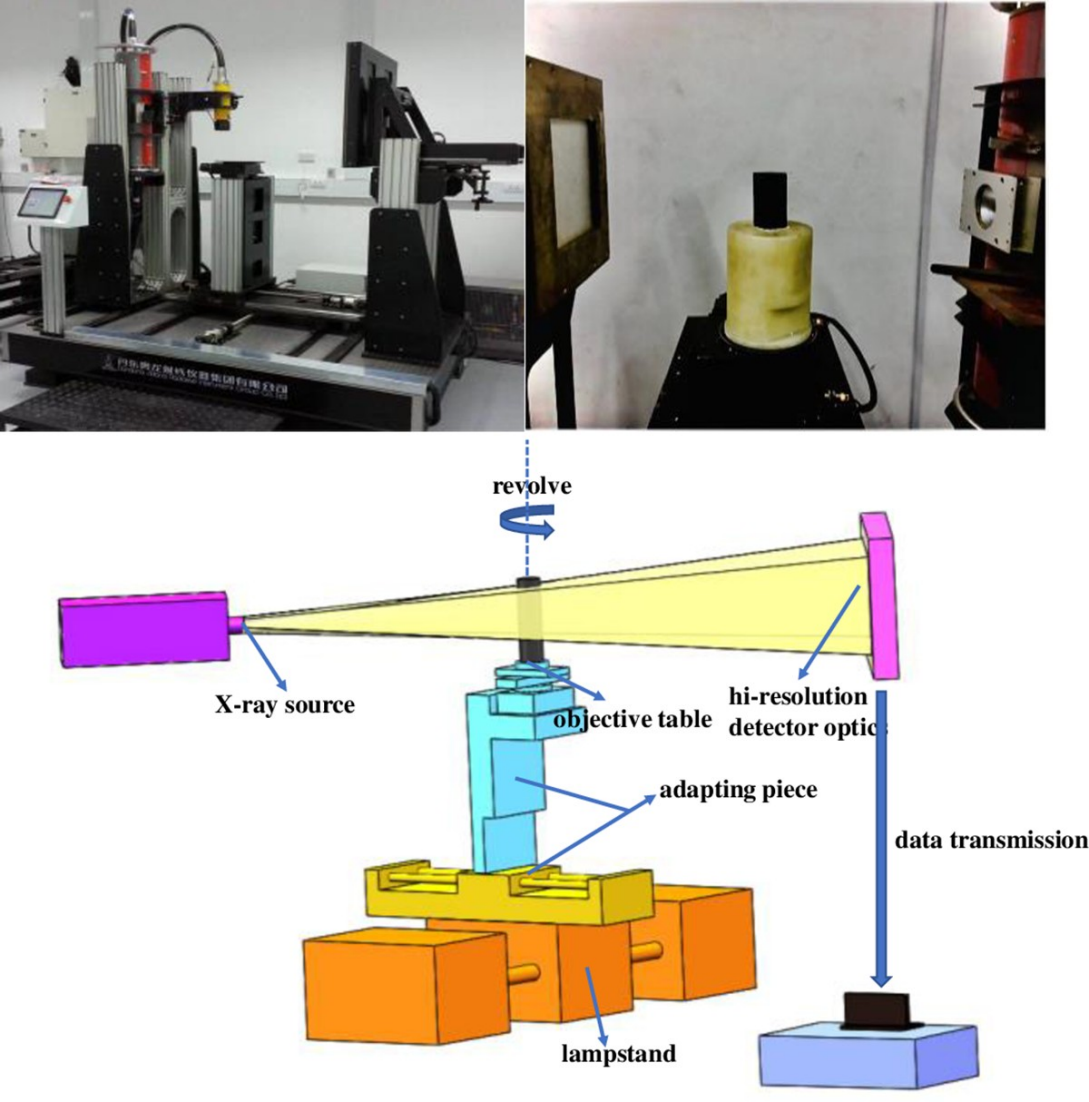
AL-CT-225 type CT scanning device.
